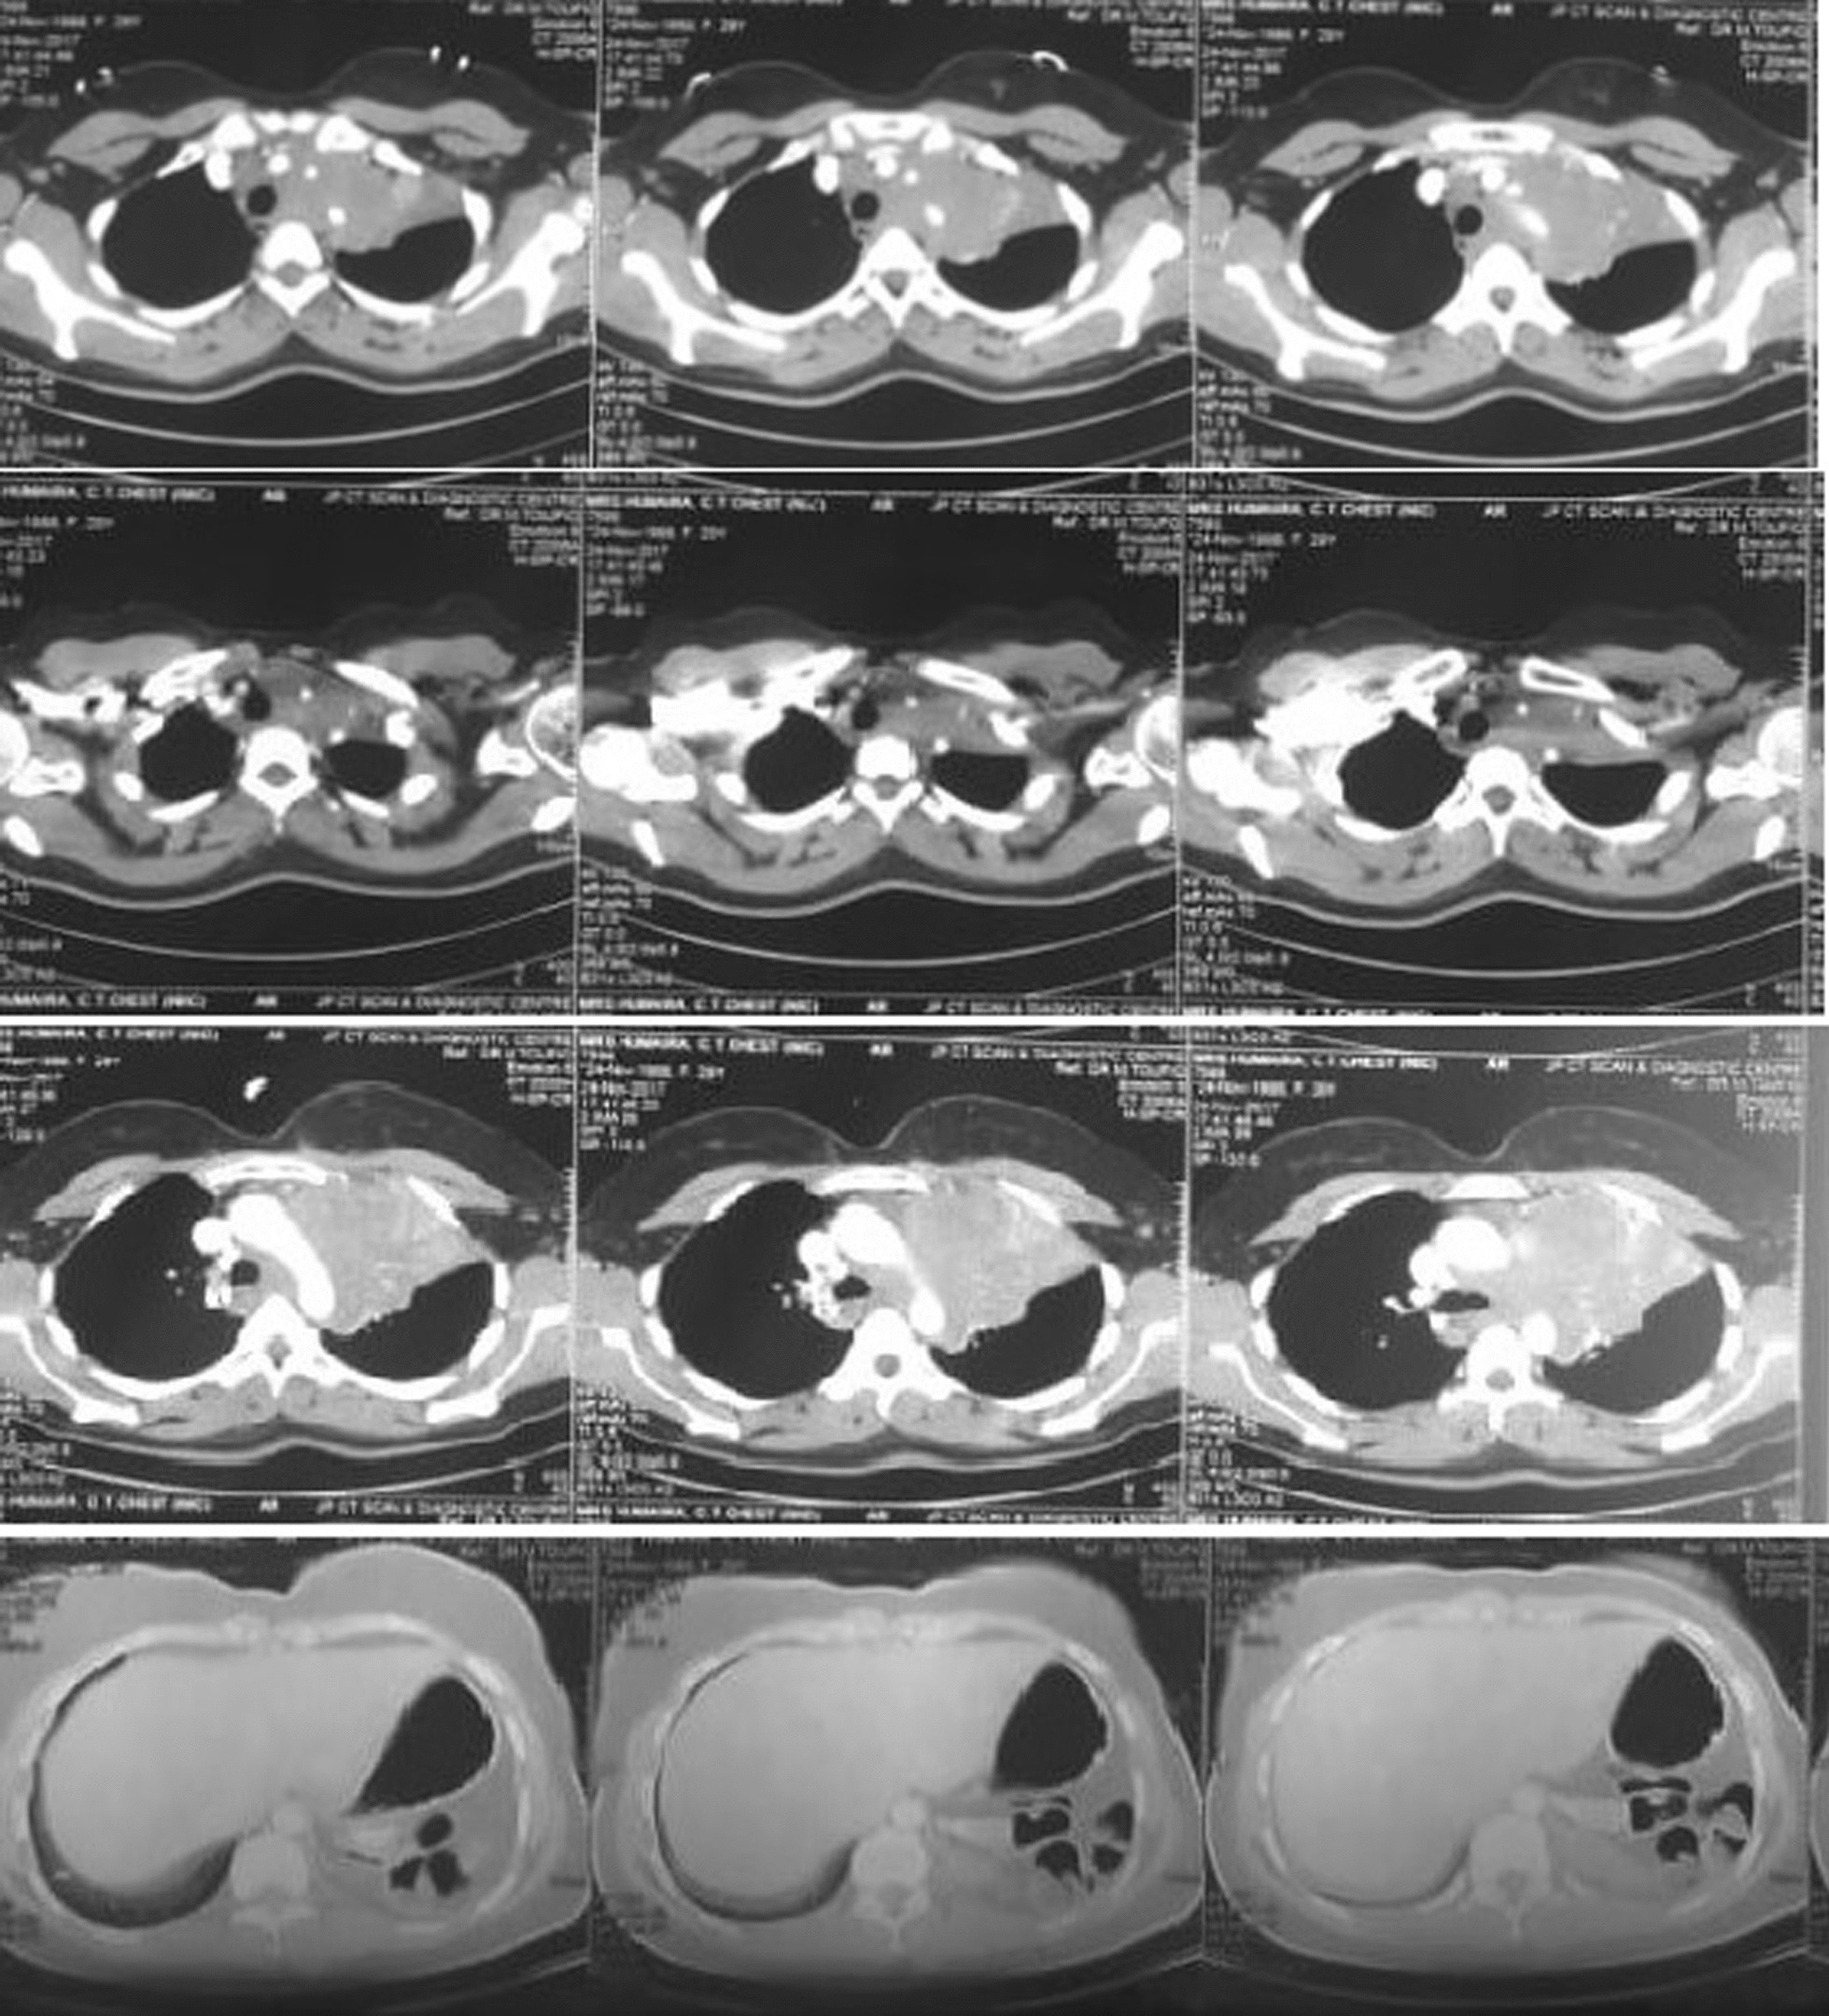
Cross sectional image of computed tomography scan of the chest showing an ill-defined hypodense lesion in the left upper lobe of lung
